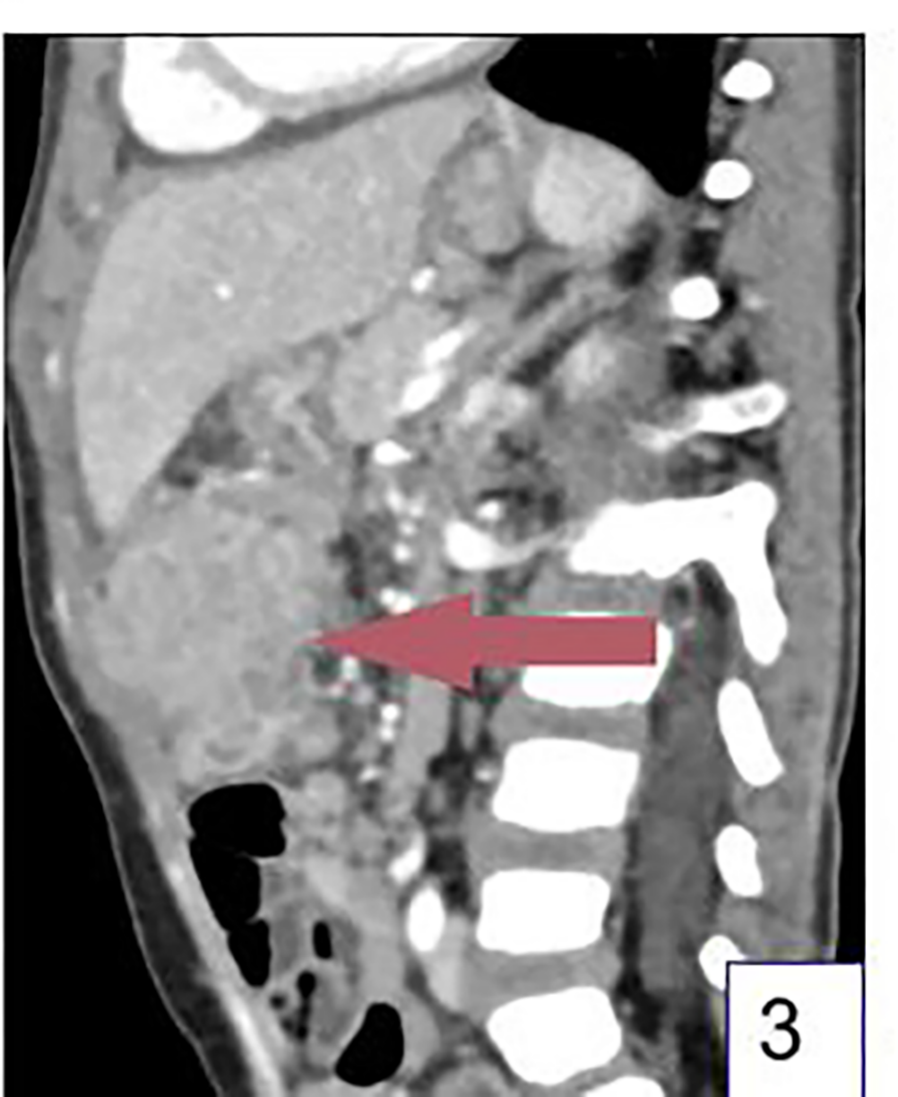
Sagittal CT image. (Arrow shows the mass).
